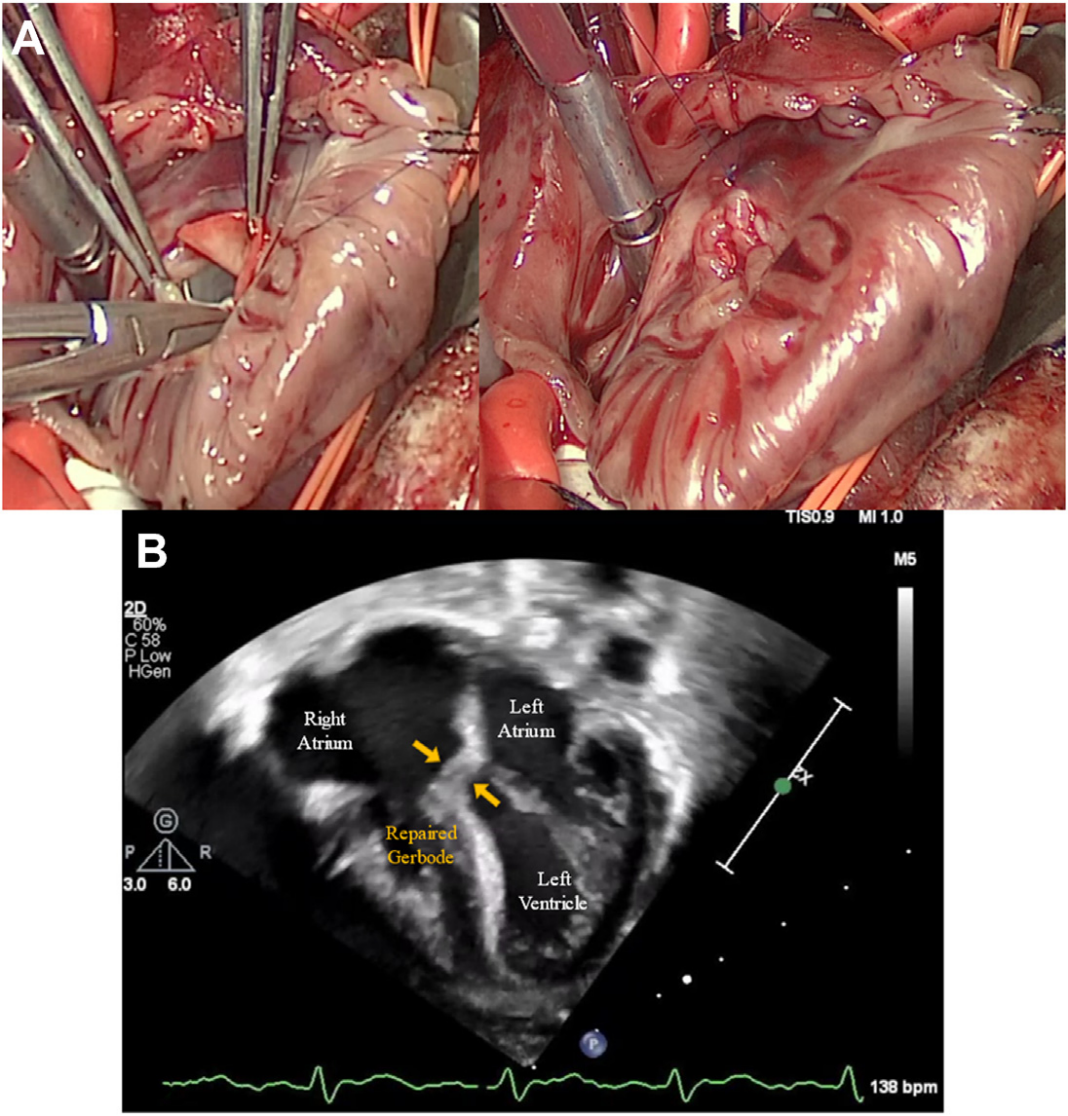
(A) Intraoperative picture of the Gerbode defect repair. (B) Postoperative transthoracic echocardiogram of repaired Gerbode defect (arrows).
